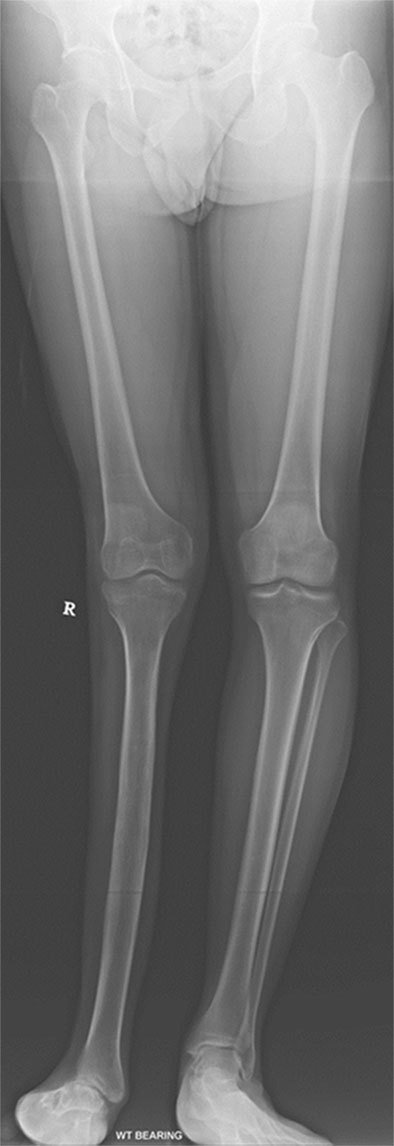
Full-length radiograph of the lower limb shows complete absence of the right fibula with genu valgus deformity of the right knee.
